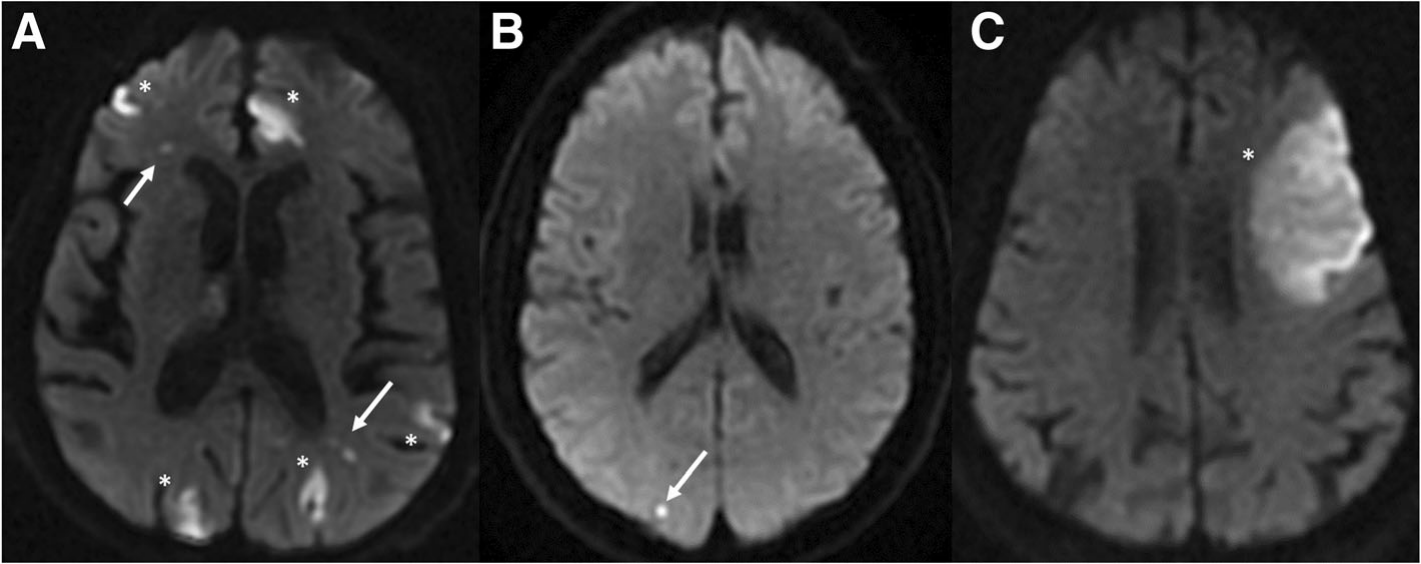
a Axial DWI obtained in a 69-year-old man two days after cardiac surgery shows multiple small (arrow) and not small (*) ischemic strokes in both hemispheres. The patient had bilateral < 50% internal carotid artery (ICA) stenosis. Absence of significant ICA stenosis and bilateral distribution suggest an embolic origin of the strokes. b Axial DWI obtained in a 72 year-old woman two days after cardiac surgery shows a small hyperintense area (arrow) in the right posterior cerebral artery territory, consistent with small ischemic stroke. The patient had bilateral < 50% ICA stenosis. c Axial DWI obtained in a 69-year-old man three days after cardiac surgery shows a large, hyperintense area in the left middle cerebral artery territory, consistent with not small ischemic stroke. The patient had bilateral < 50% ICA stenosis
